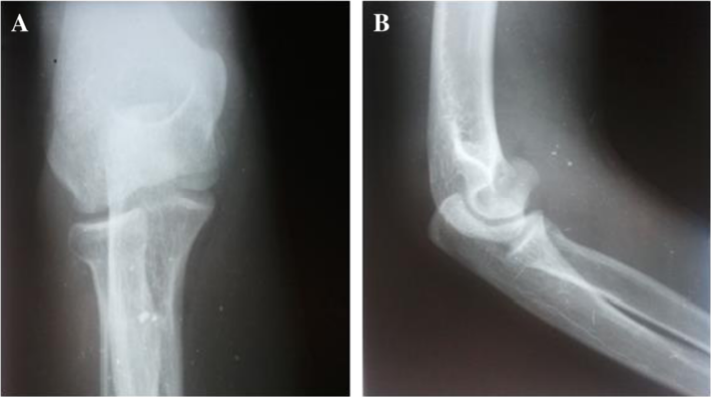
Initial radiograph. (A) Anteroposterior view and (B) lateral view.
